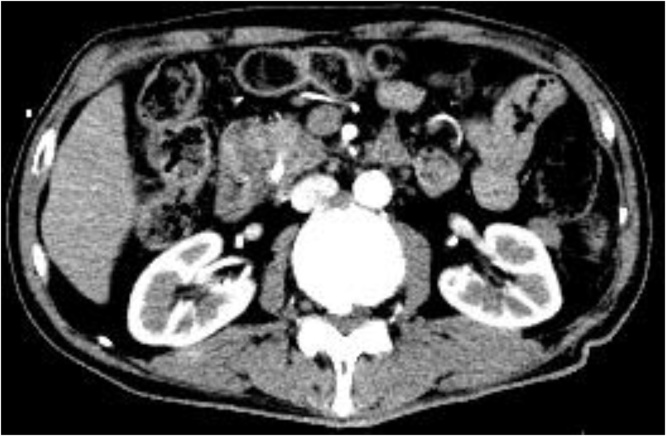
Contrast-enhanced computed tomography (CT) of the patient in case 3. Contrast-enhanced CT images revealed dilation of the intrahepatic bile duct due to a low-density tumor mass in the pancreatic head.
